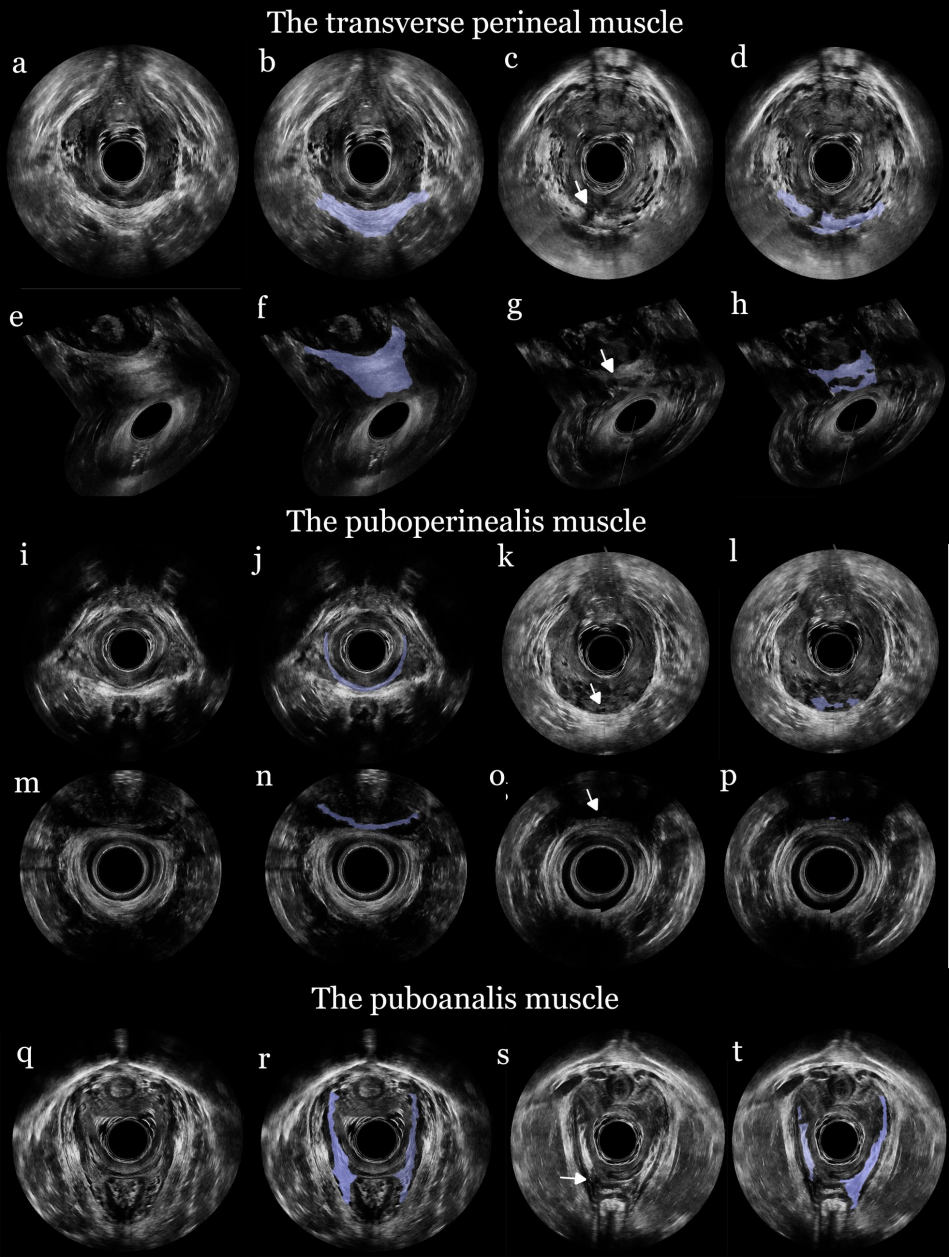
Three-dimensional ultrasound of the transverse perineal, puboperinealis, and puboanalis muscles. The transverse perineal muscle: In the three-dimensional endovaginal ultrasound in the axial plane: a Normal muscle; b Normal muscle, highlighted in blue; c An arrow pointing to a central deviation in the muscle; d Fragments of the remaining muscle, highlighted in blue. In the three-dimensional endoanal ultrasound in the coronal plane: e Normal muscle; f Normal muscle, highlighted in blue; g An arrow pointing to a central deviation in the muscle; h Fragments of the remaining muscle, highlighted in blue. The puboperinealis muscle: In the three-dimensional endovaginal ultrasound in the axial plane: i Normal muscle; j Normal muscle, highlighted in blue; k An arrow pointing to a central deviation in the muscle; l Fragments of the remaining muscle, highlighted in blue. In the three-dimensional endoanal ultrasound in the axial plane: m Normal muscle; n Normal muscle, highlighted in blue; o An arrow pointing to an absent muscle; p Fragments of the remaining muscle, highlighted in blue. The puboanalis muscle: In the three-dimensional endovaginal ultrasound in the axial plane: q Normal muscle; r Normal muscle, highlighted in blue; s An arrow pointing to a right deviation in the muscle; t Fragments of the remaining muscle, highlighted in blue
